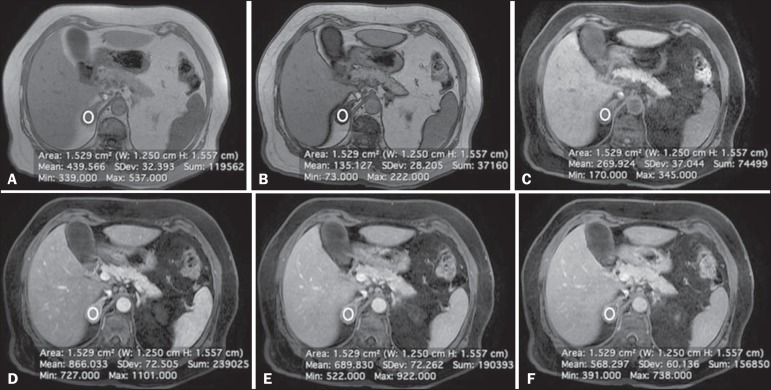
Lipid-rich adenoma in a 66-year-old woman. Transverse, T1-weighted
